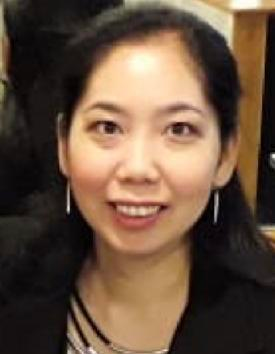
Diana Isabel Katekaru-Tokeshi is a medical doctor graduate from the National Major San Marcos University (Lima, Perú). She got a Cardiology Diploma from the Residency Programme at Hospital Militar Central (Lima, Perú) and fellowship in Cardiac Computed Tomography and Cardiac Magnetic Resonance at “Instituto Nacional de Cardiologia Ignacio Chavez” (Mexico). Now works as attending cardiologist at Hospital Nacional Dos de Mayo (Lima, Perú). Her main fields of interest are congenital heart and ischaemic heart disease.
